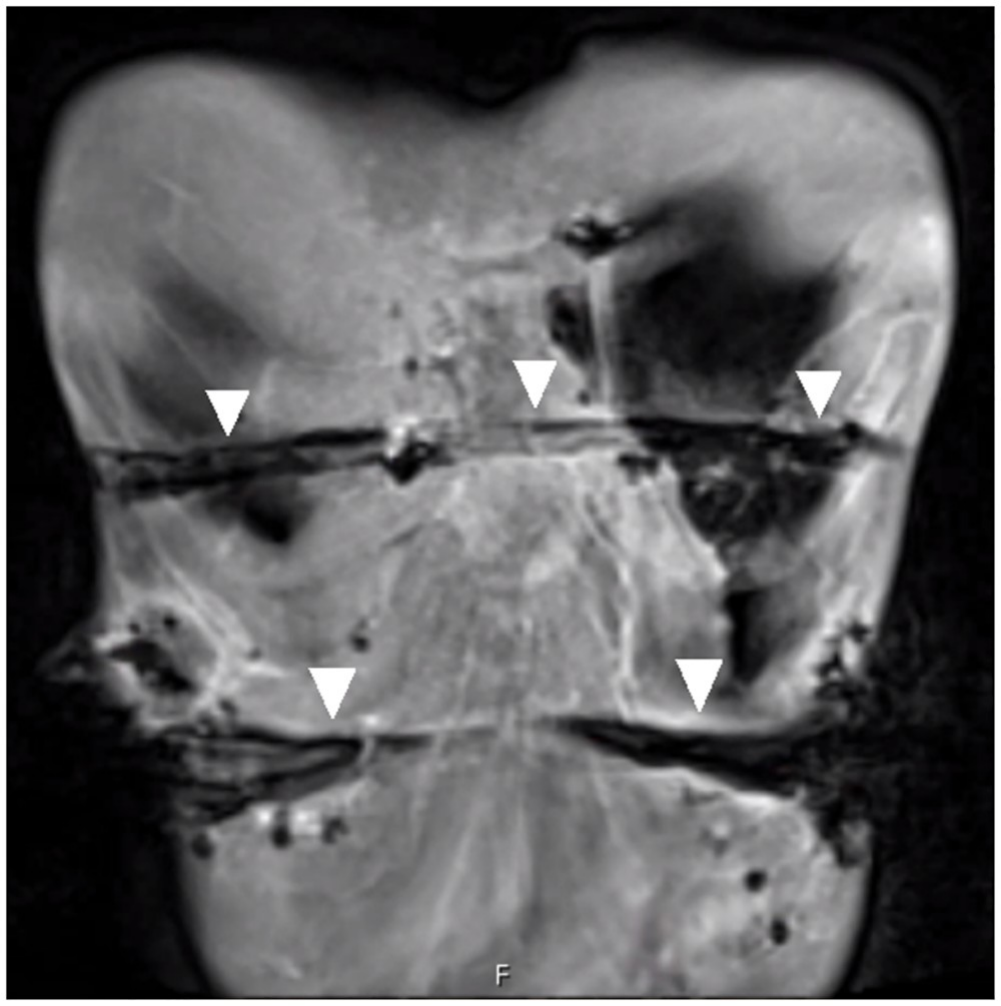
Thoracic MRI three months after removal of two TiN coated pectus bars. Coronal T1-weighted fat saturated 3D volume interpolated breath-hold magnetic resonance examination sequence after intravenous application of contrast agent shows hypointense metal susceptibility artifacts along the former bar tunnels (arrowheads). TiN = titanium-nitride.
